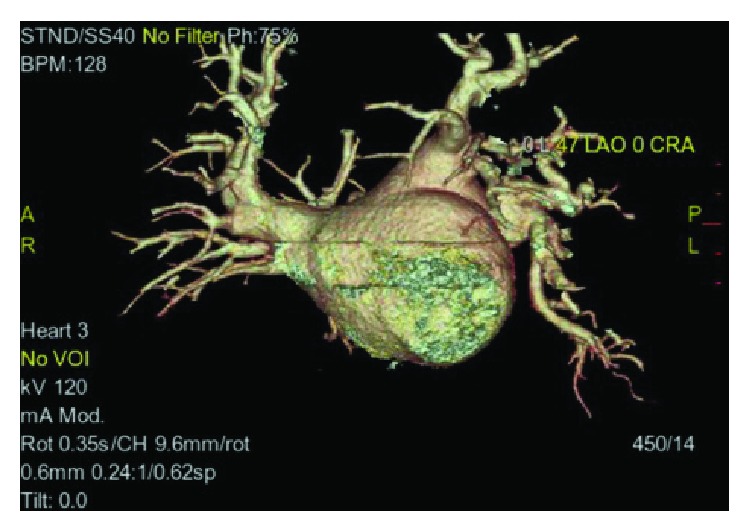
Computed tomography angiogram (CTA) of the pulmonary veins at 47 degrees left anterior oblique (LAO) view showing there is no left atrial appendage on the left atrium.
